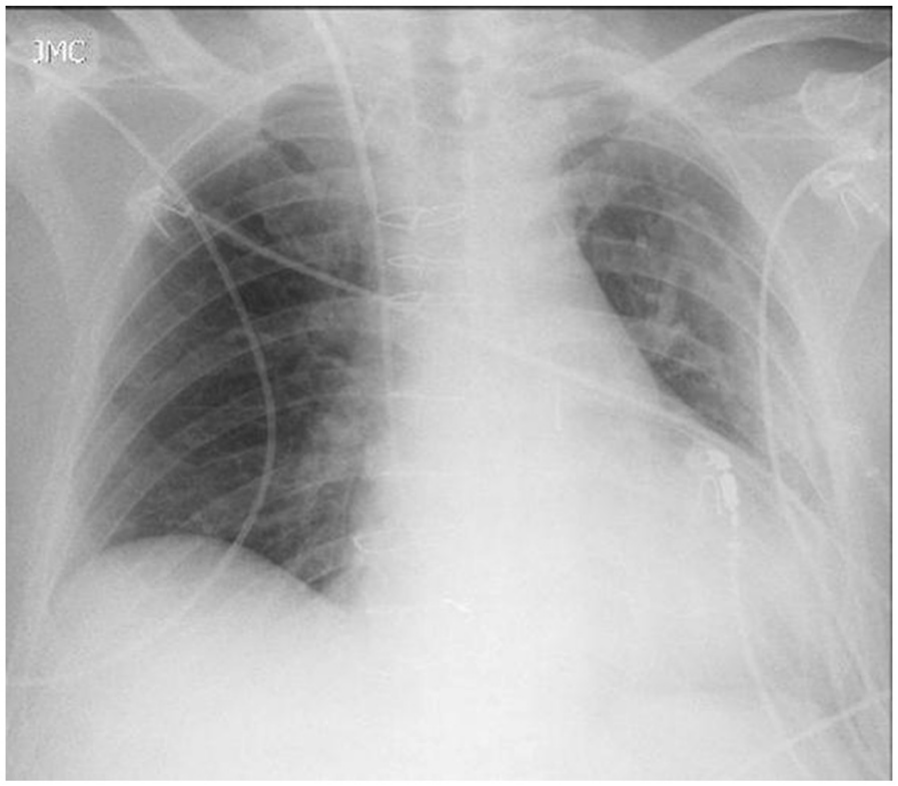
X-Ray after extubation remained without atelectasis.
